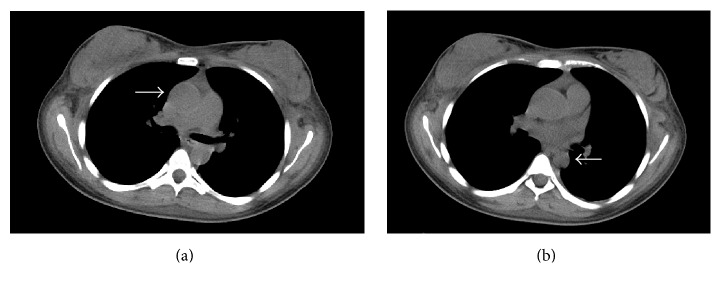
Noncontrast CT of the chest demonstrated (a) ectasia of the ascending aorta, measuring 3.4 cm, and (b) focal narrowing of the descending aorta, measuring 1.7 cm, raising concern for an aortitis in the setting of glomerulonephritis. The white arrow in (a) represents the ascending thoracic aorta and in (b) the descending thoracic aorta.
